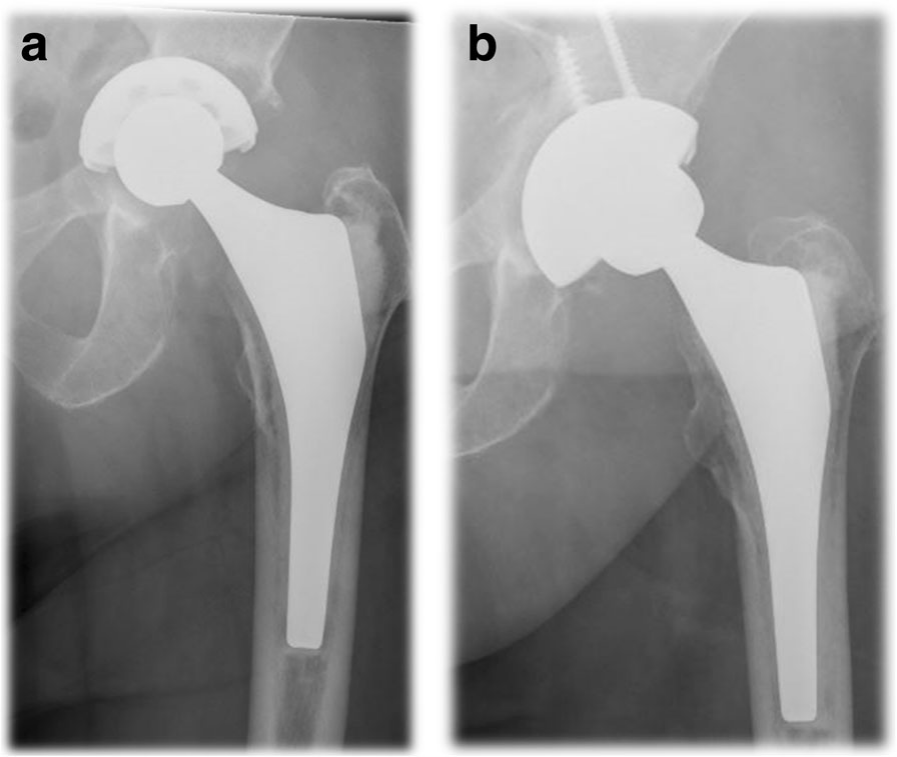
a Preoperative anteroposterior radiograph in an 81-year-old patient with aseptic cup loosening. b Anteroposterior radiograph of the same patient 5 years postoperatively, with a trabecular metal revision shell
